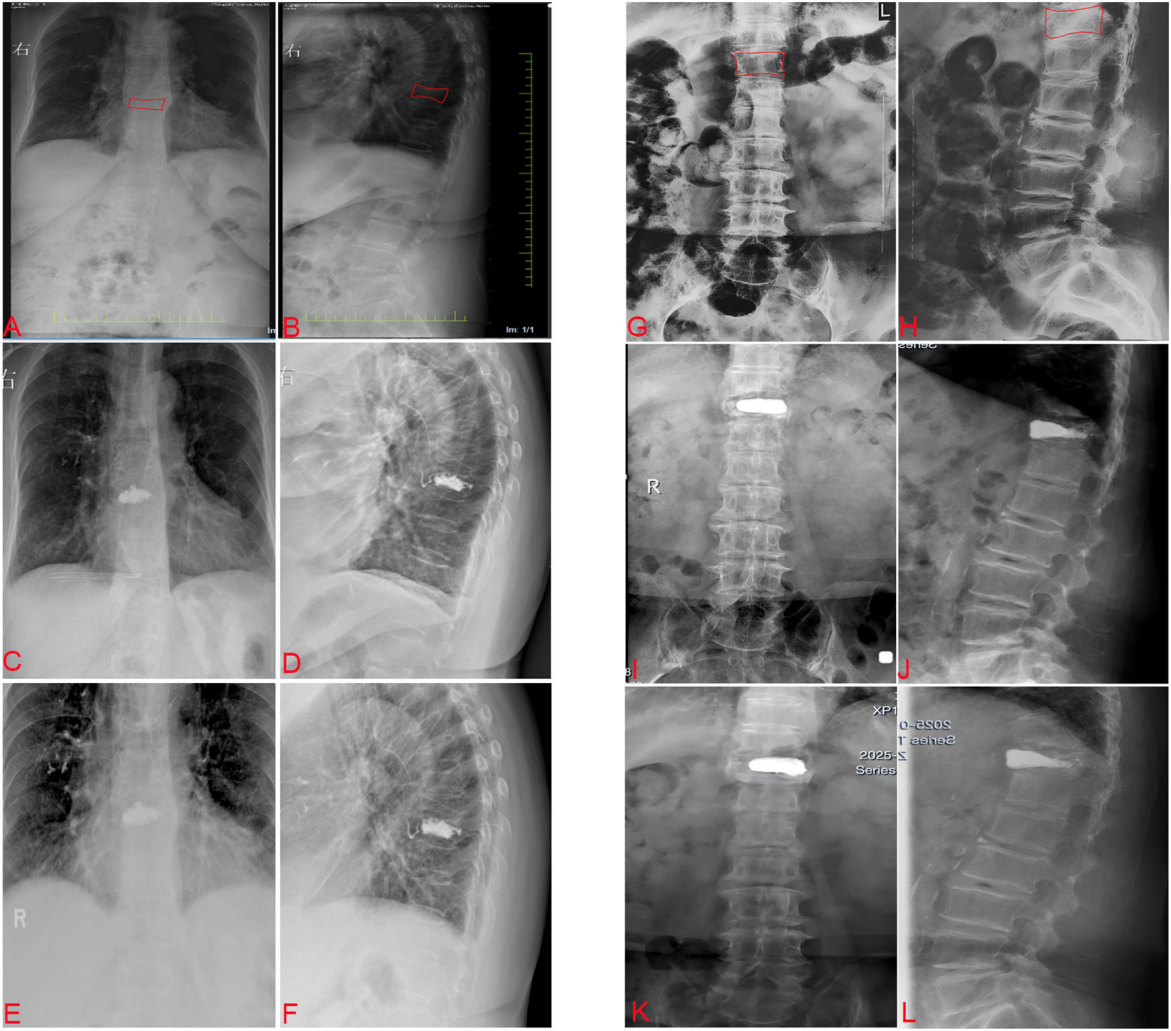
Comparison of typical cases between PVP and PKP. PVP (A-F) 67Y, F, T9 OVCF. (A) Preoperative anteroposterior X-ray view of the thoracolumbar spine. (B) Preoperative sagittal X-ray view of the thoracolumbar spine. (C) Anteroposterior X-ray view of the thoracolumbar spine at 6 months postoperatively. (D) Sagittal X-ray view of the thoracolumbar spine at 6 months postoperatively. (E) Anteroposterior X-ray view of the thoracolumbar spine at 2 years postoperatively. (F) Sagittal X-ray view of the thoracolumbar spine at 2 years postoperatively. PKP (G-L) 71Y, F, T11 OVCF. (G) Preoperative anteroposterior X-ray view of the thoracolumbar spine. (H) Preoperative sagittal X-ray view of the thoracolumbar spine. (I) Anteroposterior X-ray view of the thoracolumbar spine at 6 months postoperatively. (J) Sagittal X-ray view of the thoracolumbar spine at 6 months postoperatively. (K) Anteroposterior X-ray view of the thoracolumbar spine at 2 years postoperatively. (L) Sagittal X-ray view of the thoracolumbar spine at 2 years postoperatively. PVP, percutaneous vertebroplasty, PKP, percutaneous kyphoplasty, OVCF, osteoporotic vertebral compression fractures.
